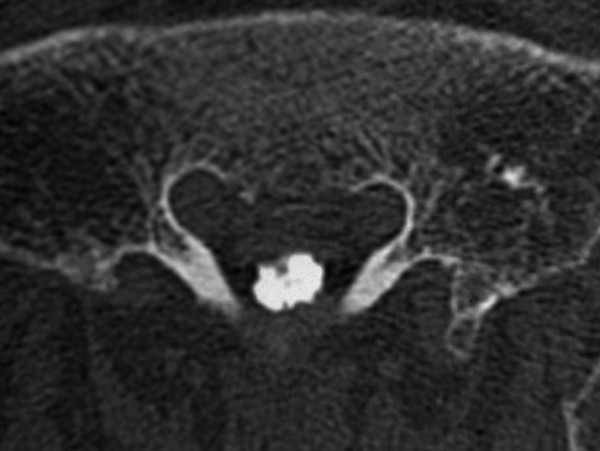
Myelo-computed tomography showed irregular images in the dural sac wall from S1 level to the most caudal region of the dural sac.
